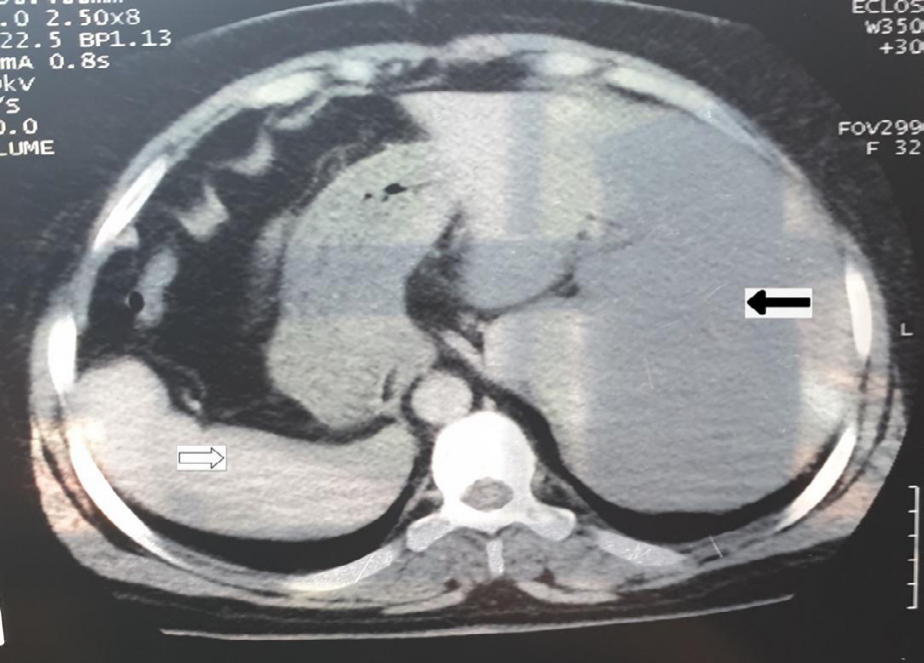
CT abdomen showing liver on left hypochondrium (solid arrow), and spleen on right hypochondrium (empty arrow).
